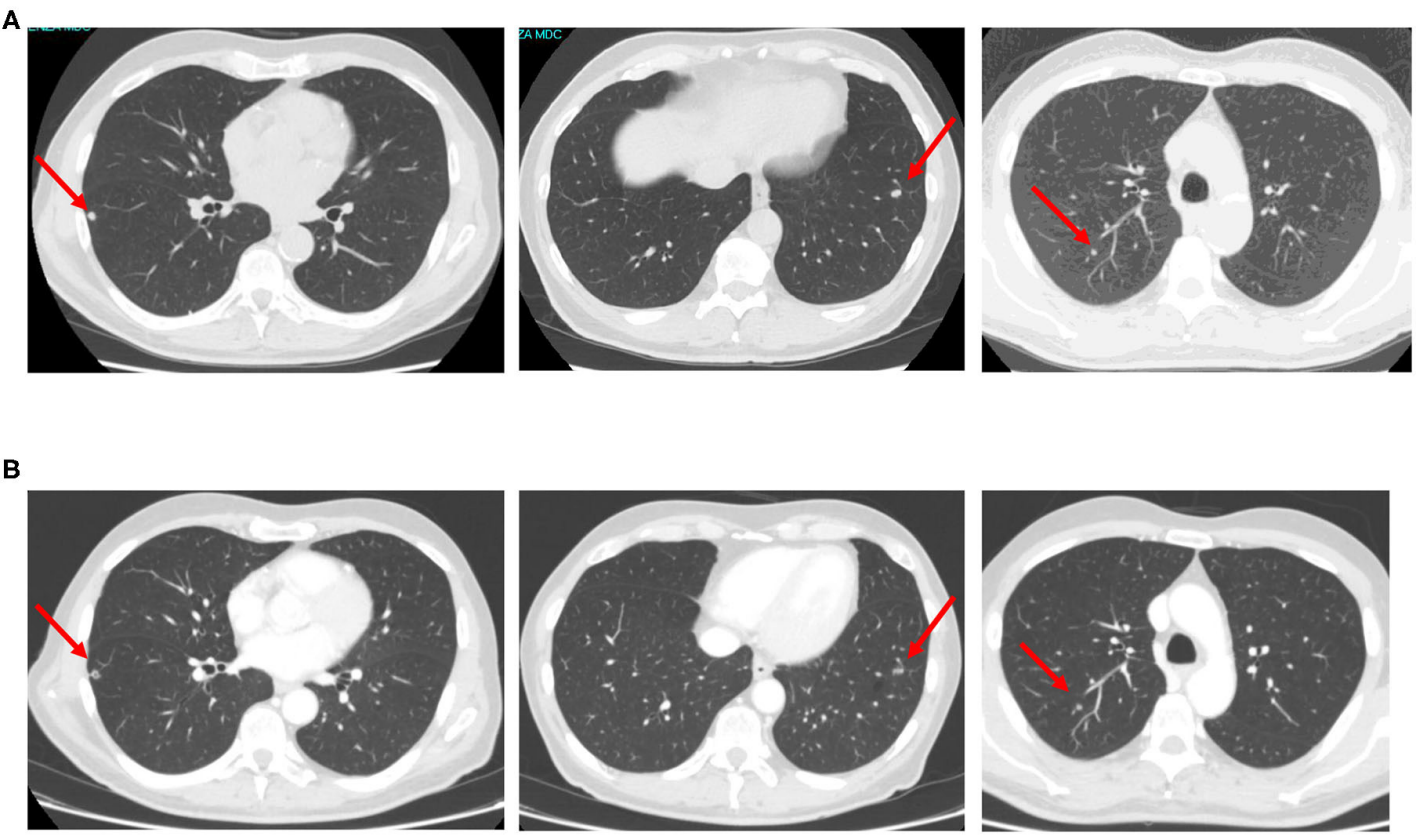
CT scan images showing bilateral lung metastases early relapsed after adjuvant chemotherapy (A) and re-evaluated after first-line aflibercept/chemotherapy (B).
